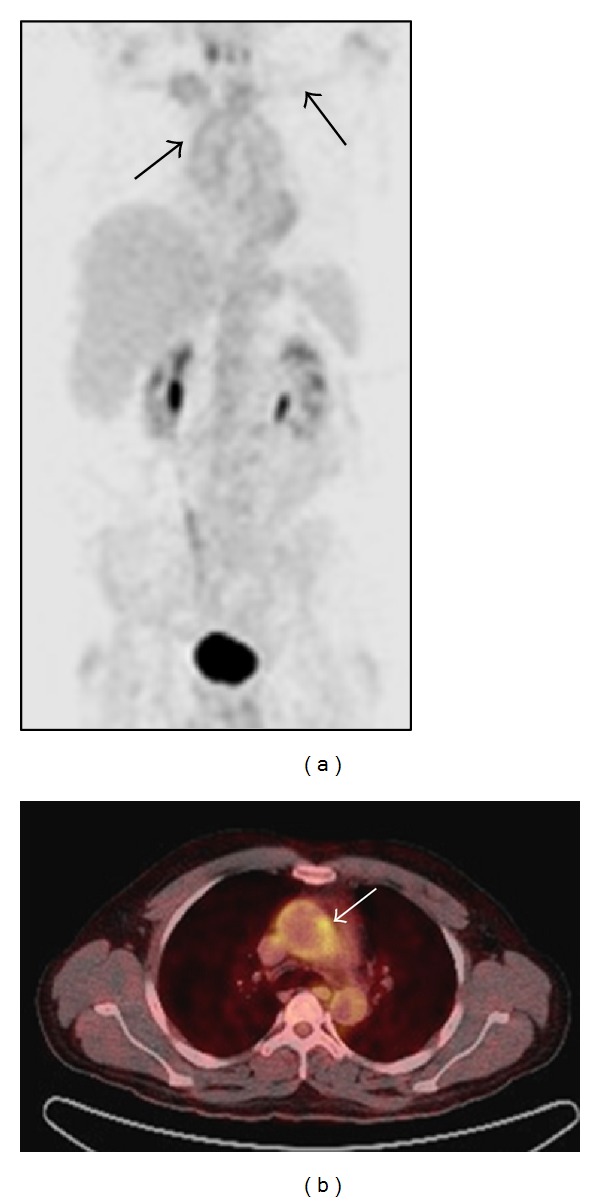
Fluorodeoxyglucose positron emission tomography. (a) An ^18^F-FDG-PET scan in a young woman with active Takayasu arteritis demonstrating homogeneous linear uptake of FDG in the arch of the aorta and the subclavian arteries, suggestive of active disease. (b) Coregistered ^18^F-FDG-PET-CT image demonstrating increased uptake localised to the wall of the thoracic aorta of a man of 26 years with active disease.
